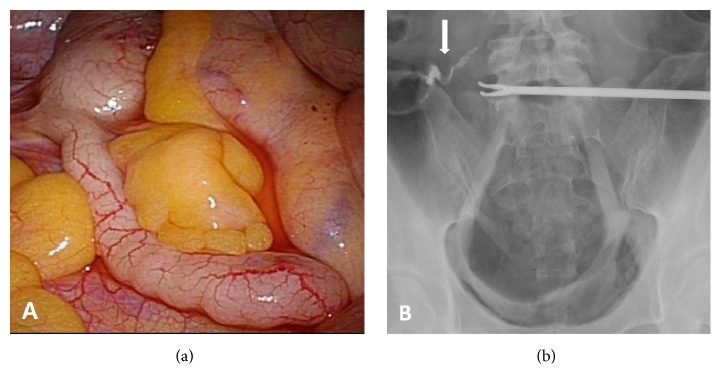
Examination by relaparoscopy. (a) Perioperative photo. No remnant was found where the cord-like material was assumed to exist. (b) Perioperative X-ray test. The shape and position of the material were found to be coincident with the appendix in (a).
